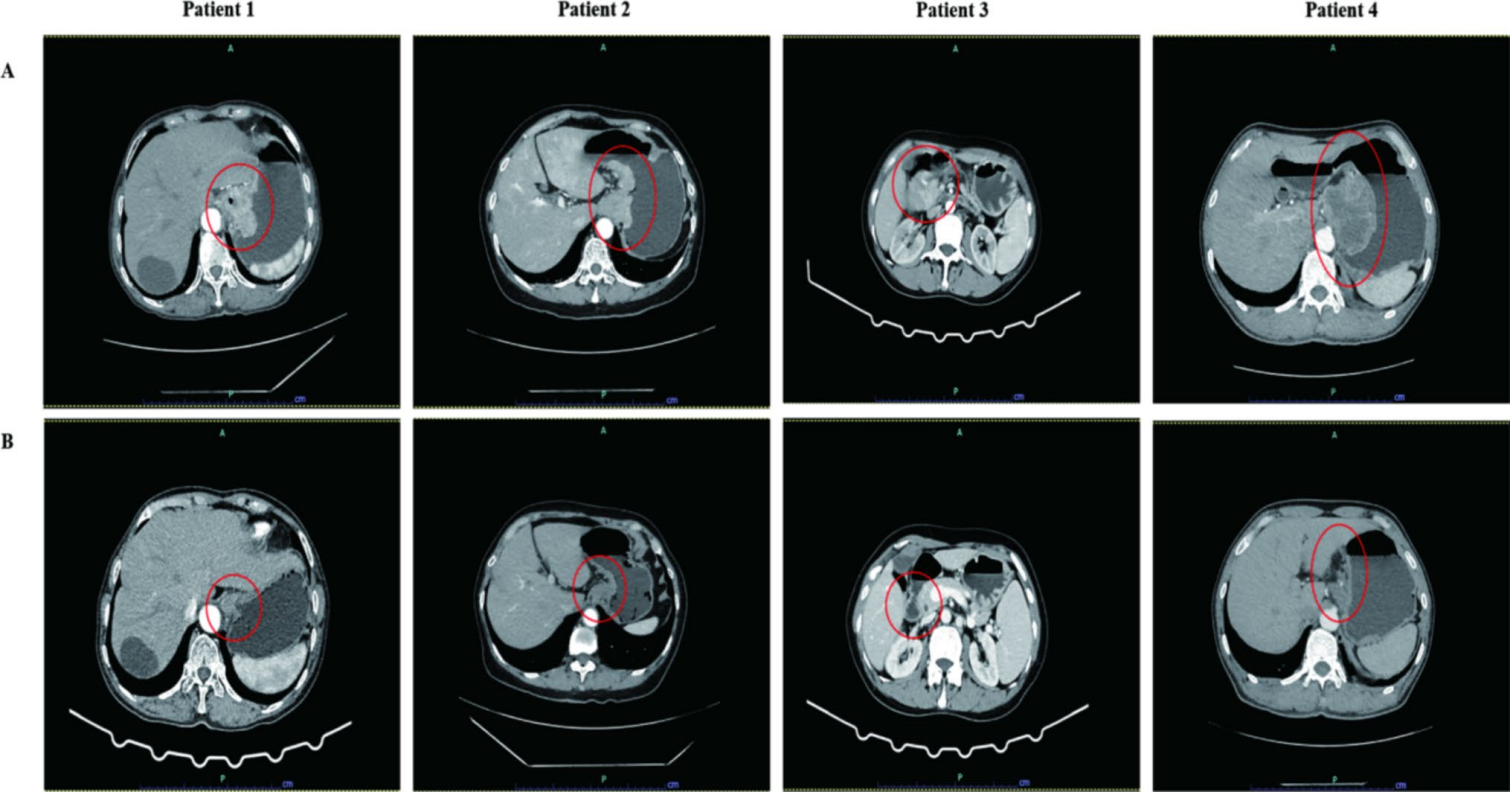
Abdominal CT scans demonstrating the disease progression following treatment. (a) CT scans at the diagnosis (before neoadjuvant therapy). (b) reexamination of CT scans before surgery (after neoadjuvant therapy). The red circle points out the location of the tumor. CT: Computed tomography
